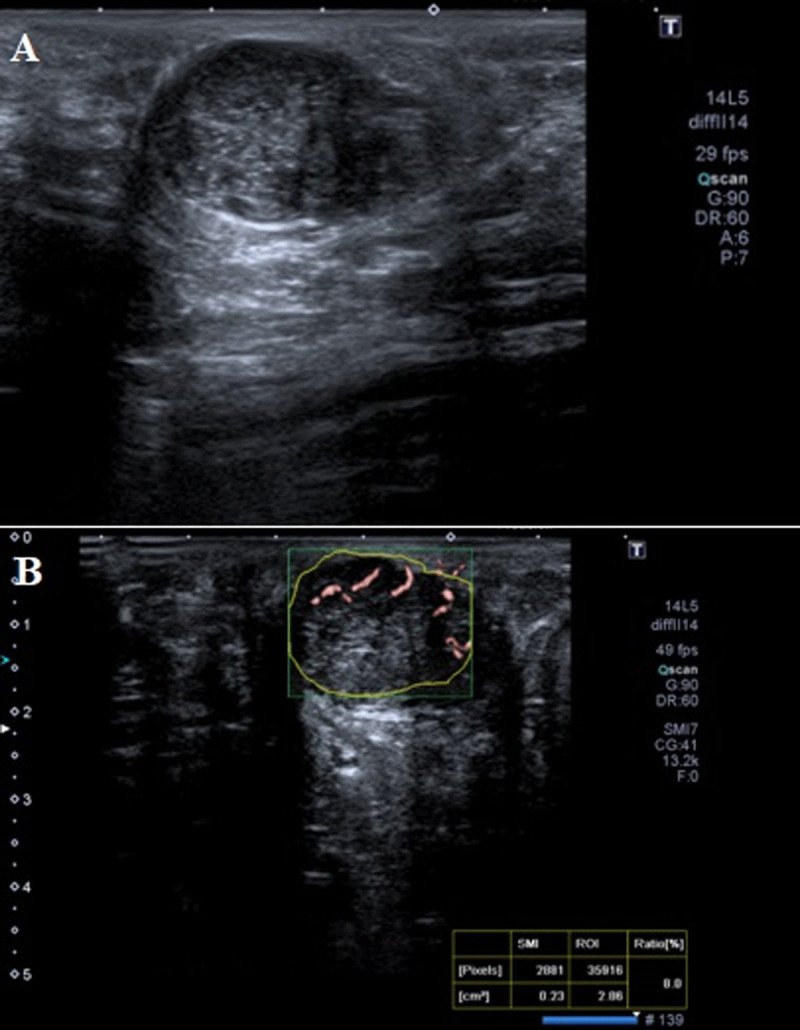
Magnetic resonance imaging (MRI) revealed bilateral ectopic axillary breast tissue (*Figure 2A*, white arrows) with a T2 hyperintense nodular lesion in the left axilla (*Figure 2B*, dashed arrow). Dynamic contrast enhanced magnetic resonance imaging (DCE-MRI) showed homogeneous contrast enhancement in left axillary mass (*Figure 2C*). Fibroadenoma and lymphadenopathy were considered as differential diagnosis.
